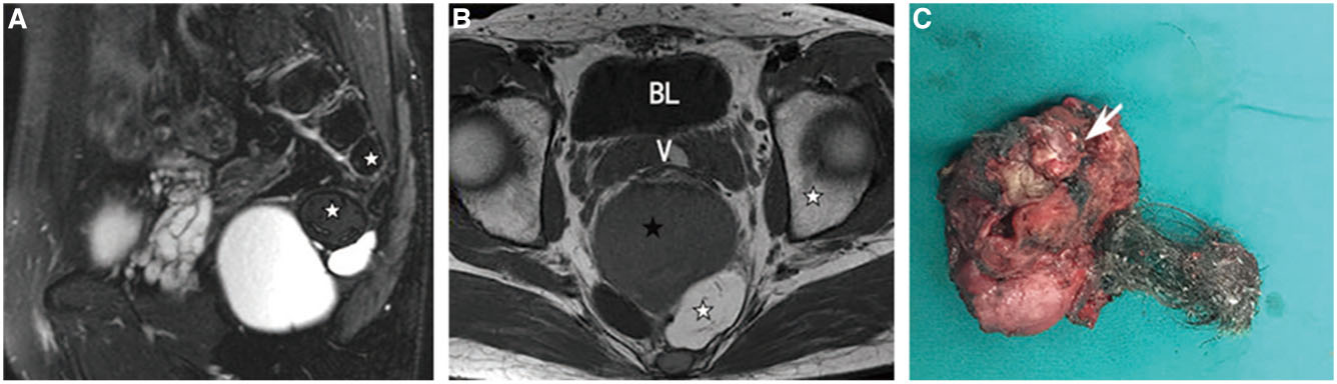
Magnetic resonance imaging of a patient with a teratoma. (A) Sagittal T2-weighted fat suppression image. (B) Axial T1-weighted image. (C) The solid components in the tumour are bony (white arrow). Homogeneous low intensity (T1W1) indicates cystic components (dark asterisks), while low intensity (T2W1-FS) or inhomogeneous slightly high intensity (T1W1) indicates solid components (white asterisks), which were confirmed as bony or calcified. BL, bladder; V, seminal vesicle.
